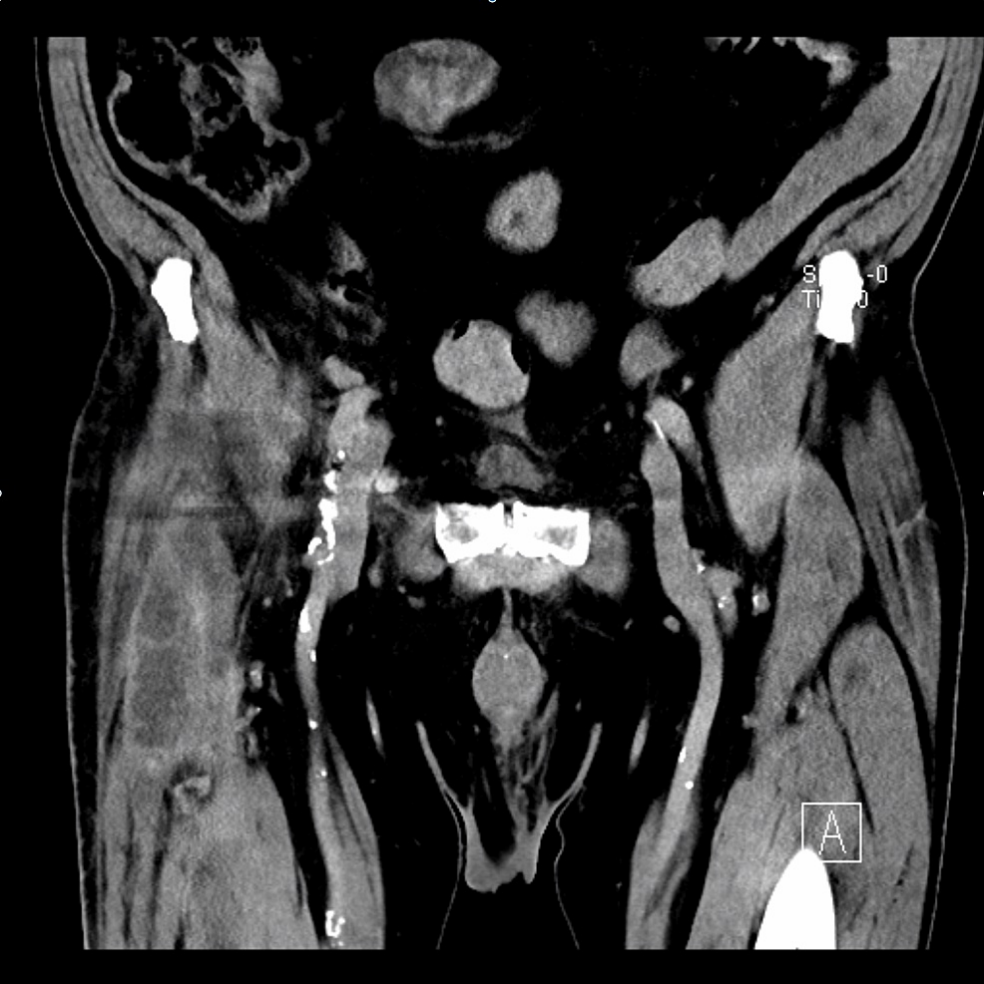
Preoperative contrast-enhanced CT scan of the periprosthetic fluid collection CT, computed tomography
